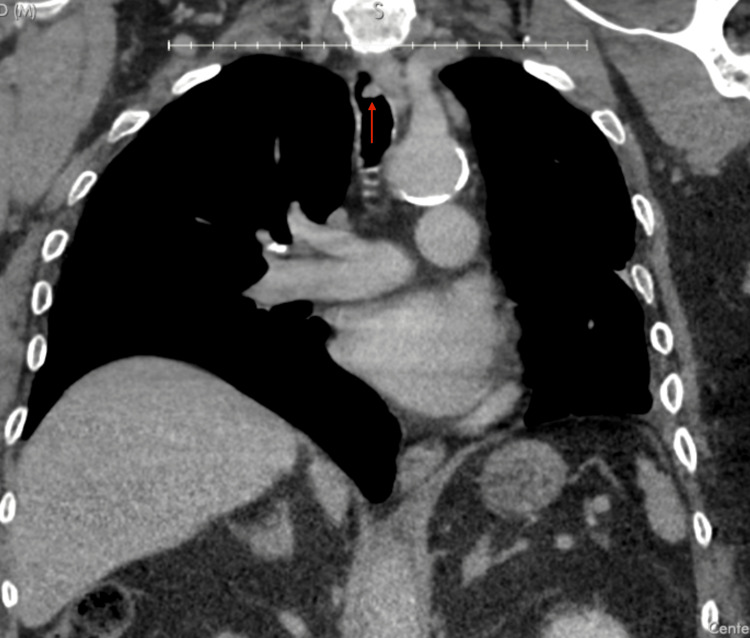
Coronal view of the patient's noncontrast CT chest imaging. A metastatic tracheal lesion (red arrow) is demonstrated. The left mainstem bronchus lesion was not detected CT: computed tomography
